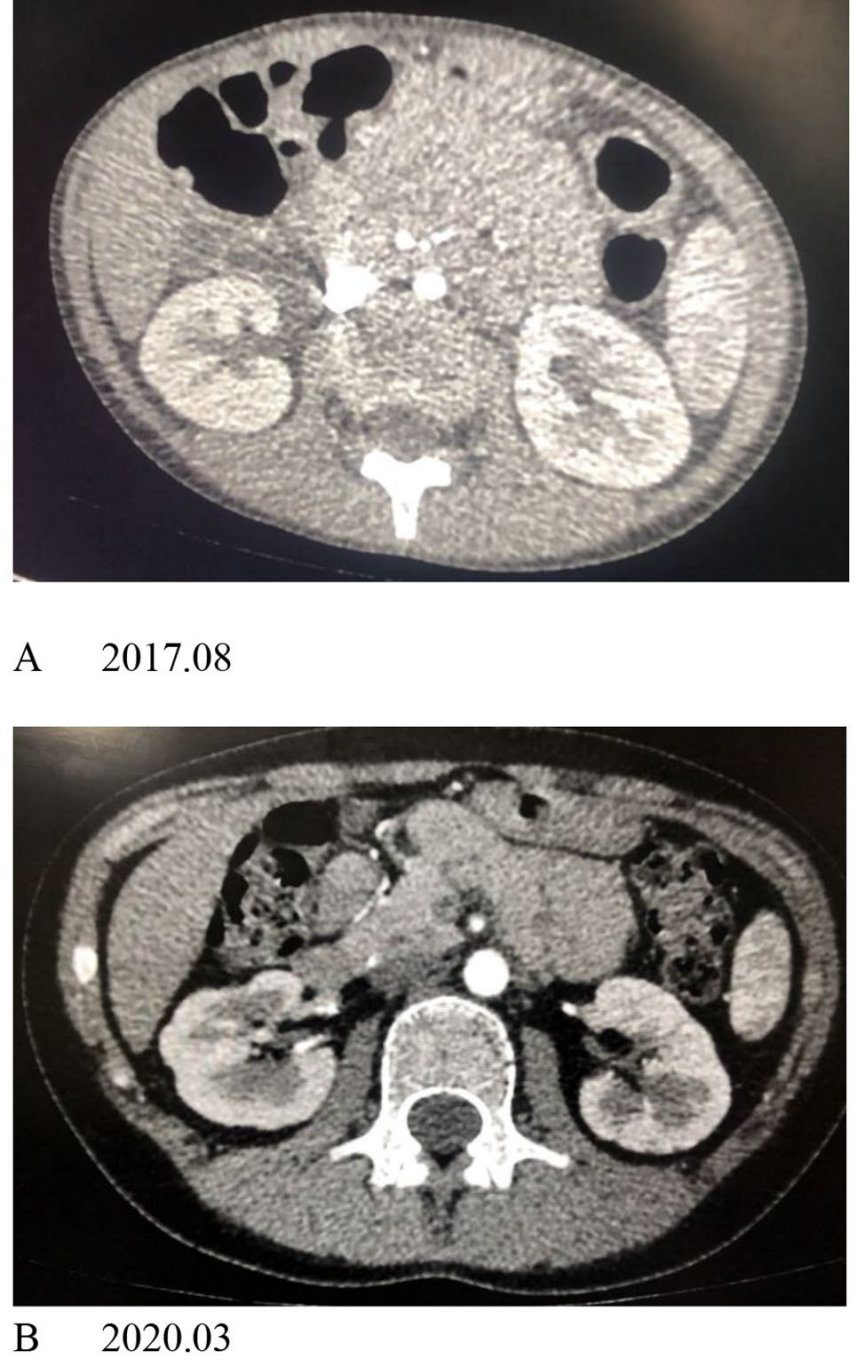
Patient 3, a 12-year-old girl, underwent axial contrast-enhanced CT imaging. A showeds accessory renal artery stenosis of the right kidney; the renal volume was normal with a smooth margin. B After 31 months, the edge of the right kidney became irregular due to focal thinner renal cortices
